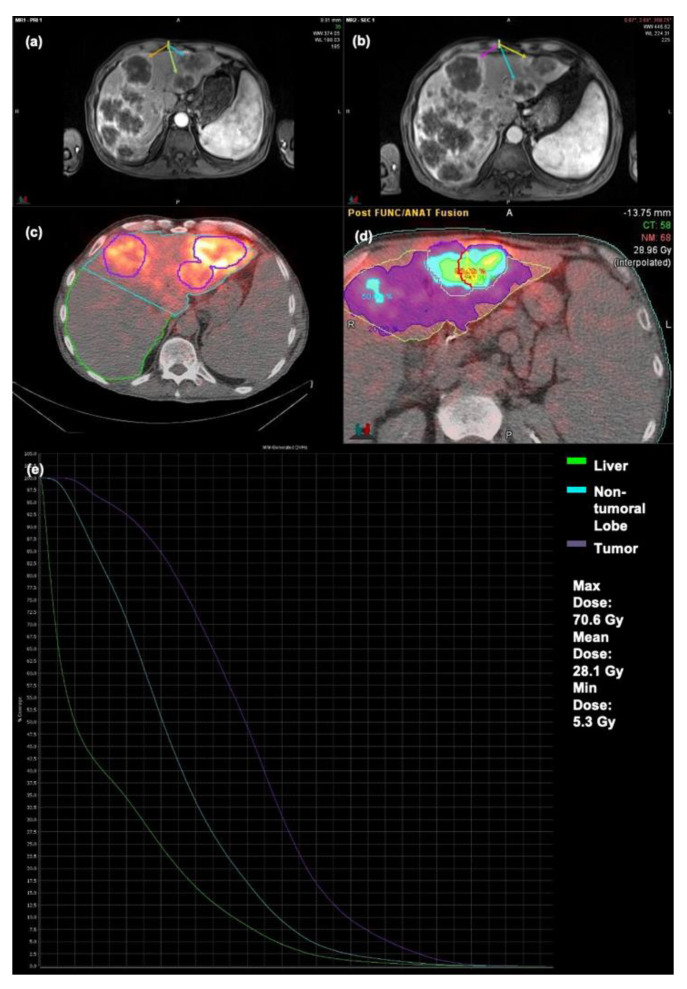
Patient failing to show OR following Y90 therapy (a) Pre-op MRI (b) Post-op MRI (c) Bremsstrahlung SPECT/CT (d) Dosimetry map (e) DVH Curve.
